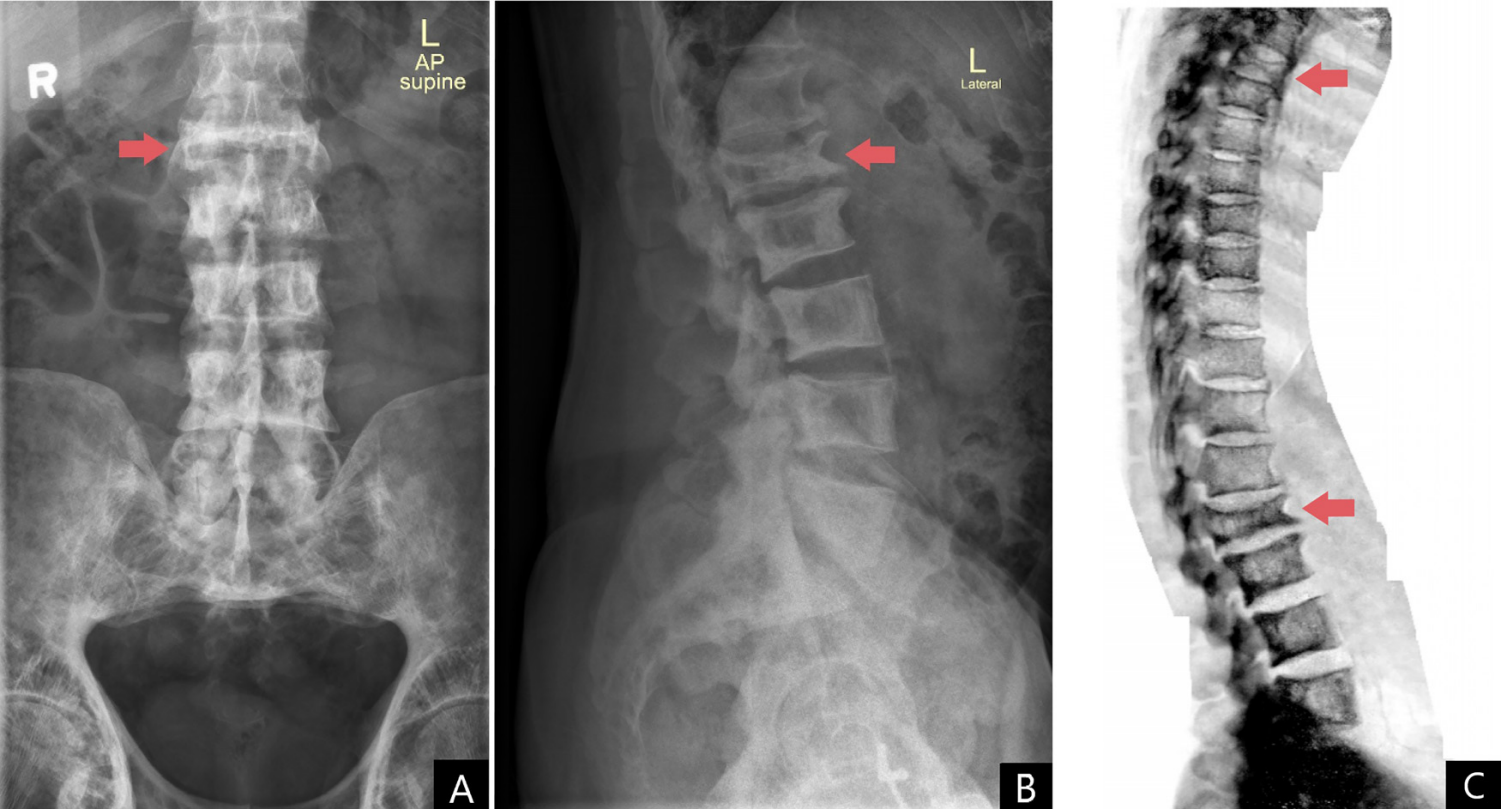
Follow-up x-rays and complications of compression fracture of T3 and L1 with lytic lesion in T12. (A) Lumbar AP view. (B) Lumbar lateral view. (C) Reconstructed full view spine. Compression fractures marked in red.
